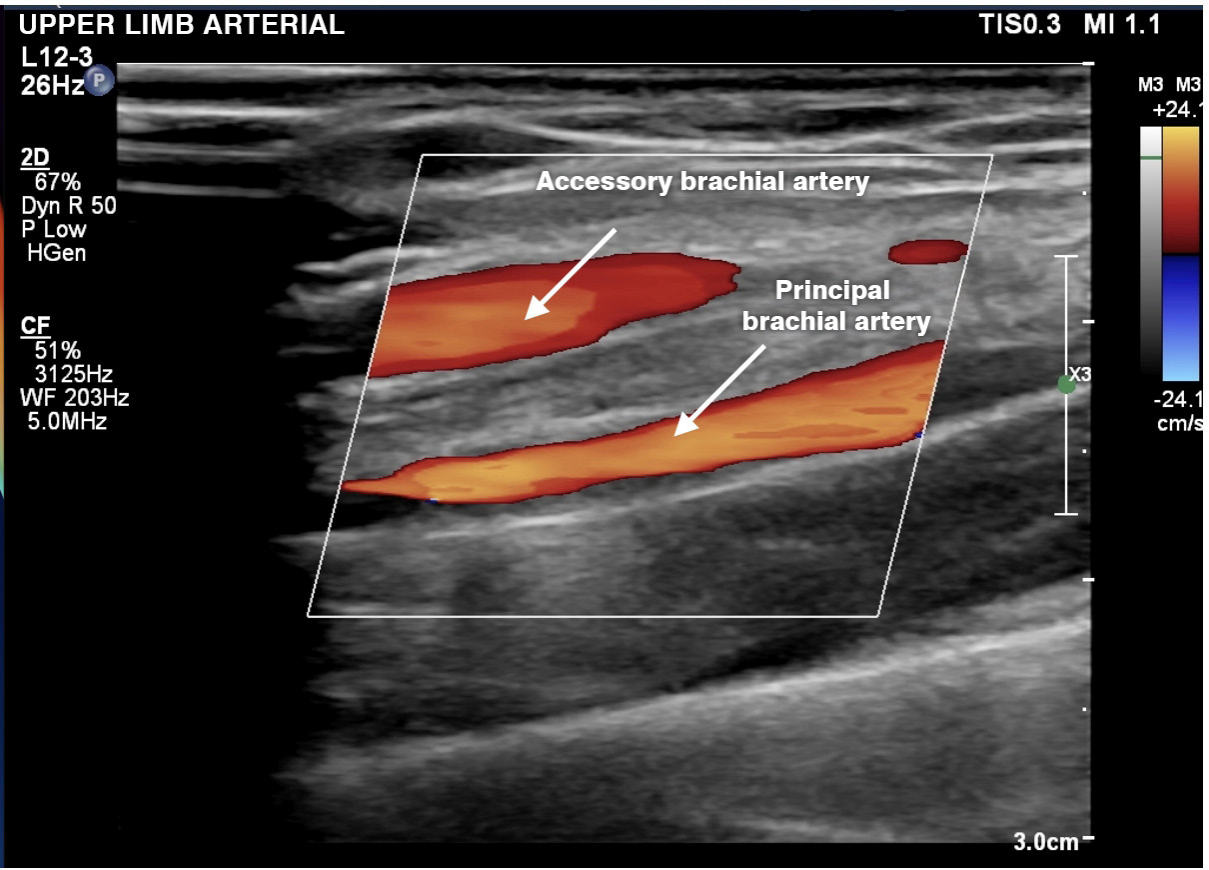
Doppler ultrasound in longitudinal view, showing an accessory brachial artery and the principal brachial artery.
